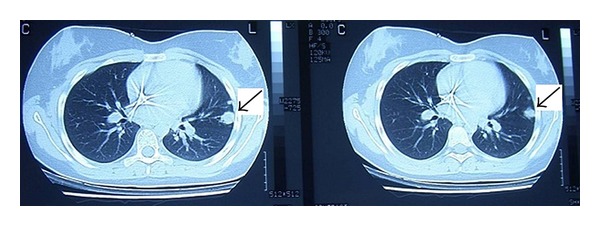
Computed tomography investigation: nodular lesions in the left lower lobe of the lung.
